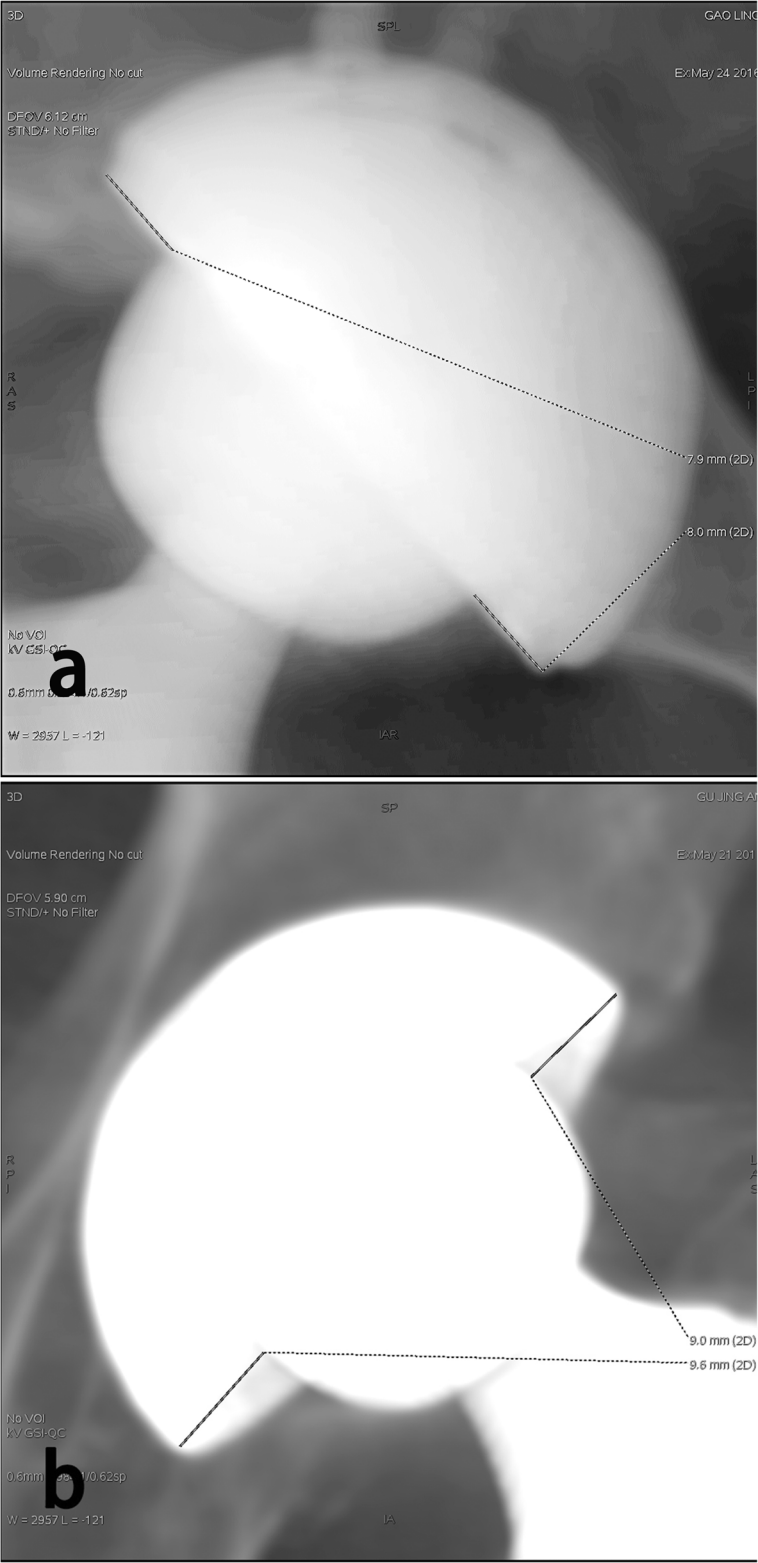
Measurement of femoral head penetration into the liners (measured on reconstructed CT). One line is drawn from the superior to the inferior edge of the acetabular component. The distances from the superior margin of the acetabular component to the femoral head and from the inferior margin of the acetabular component to the femoral head were measured. a A 37/F patient underwent THA with CoC bearing for right AVNF, the femoral head penetration was measured on reconstructed CT at postoperative 7 years. b A 69/F patient underwent THA with CoXPE bearing for left AVNF; the femoral head penetration was measured on reconstructed CT at postoperative 8 years
